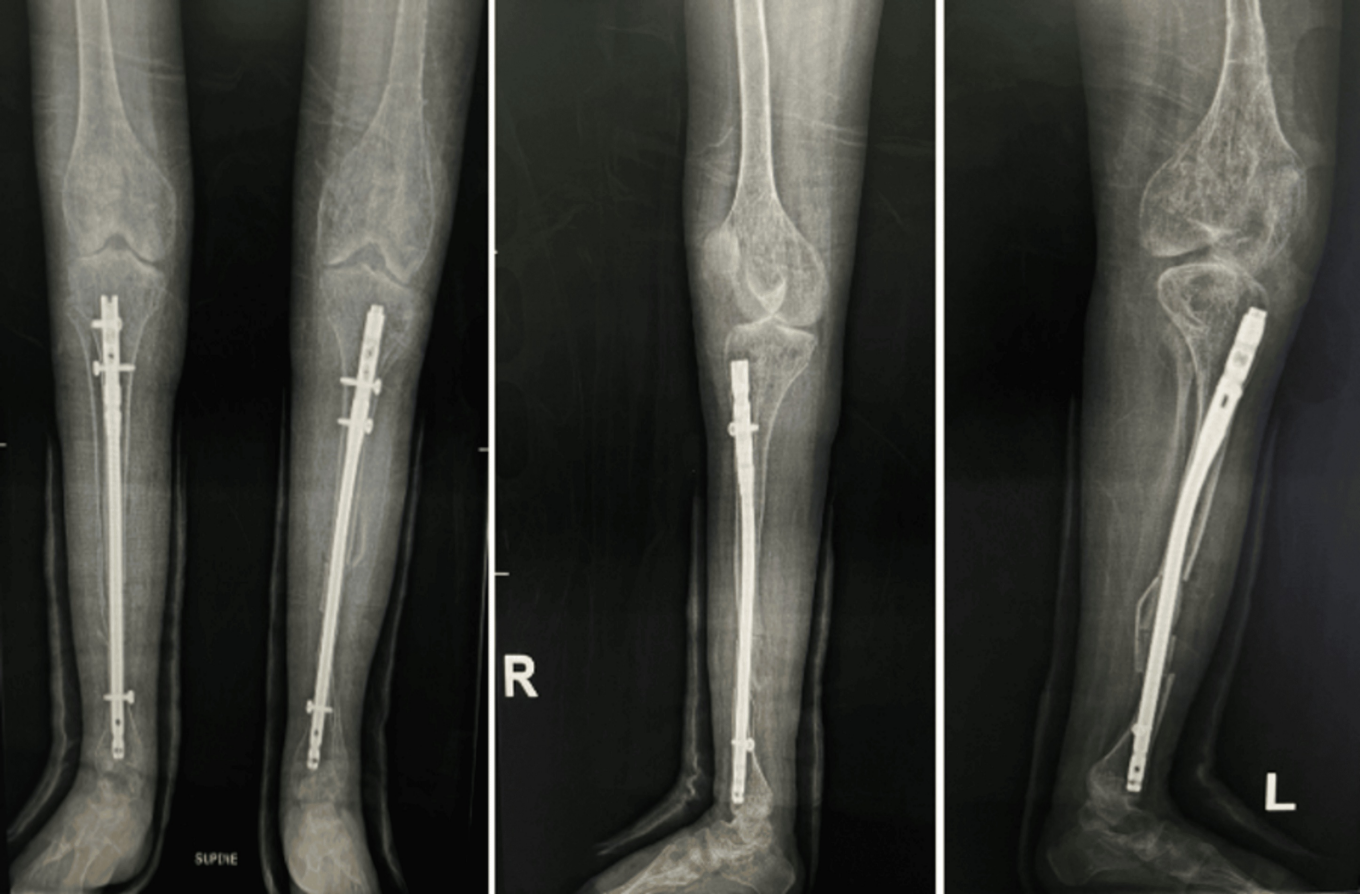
Postoperative patient X-rays Postoperative radiographs of both tibiae demonstrating intramedullary nail fixation following multiple corrective osteotomies for bilateral tibial bowing deformity. Anteroposterior and lateral views show satisfactory alignment and stable fixation of the right and left tibiae.
